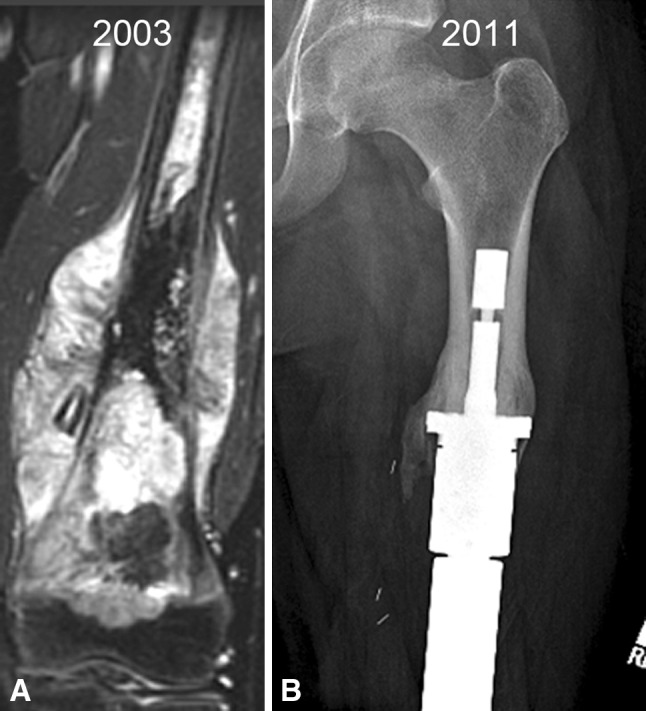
The Compress^®^ is ideally suited for situations where there is a short remaining intramedullary canal that can only accommodate a short stem and fixation would be compromised. (A) An image shows the femur of a 15-year-old patient who had resection of a 25-cm osteogenic sarcoma of the distal femur that extended into the proximal 1/3 of the diaphysis and required a 28-cm resection. (B) A radiograph demonstrates how the surrounding bone responds to the compliant force over time.
